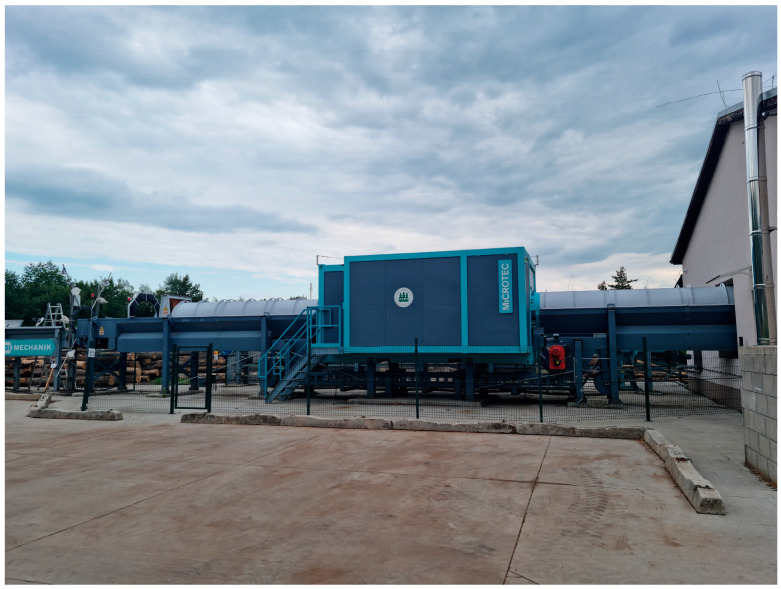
Three-dimensional CT scanner manufactured by Microtec based in Italy.
